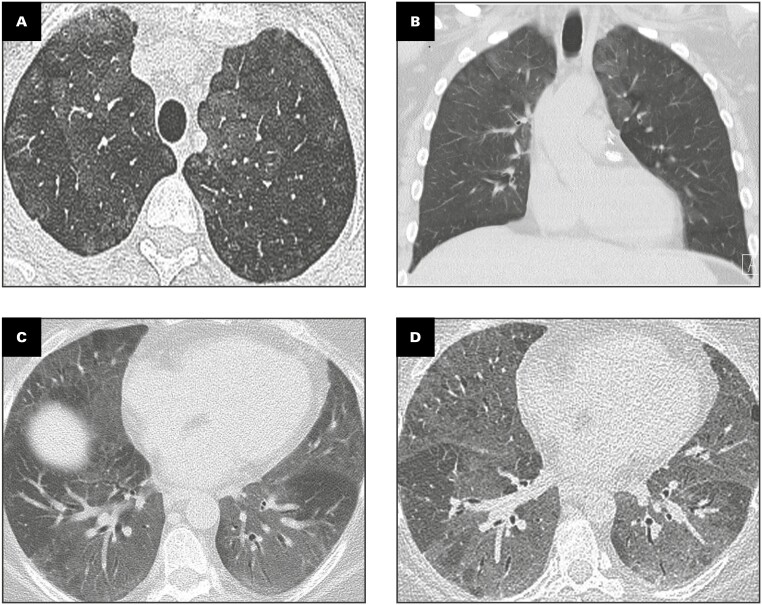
Radiologic findings in smoking-related interstitial fibrosis. A, B, Case 2 (44-year-old woman, 15 pack-year current smoker). High-resolution axial computed tomography (CT) (A) and nonenhanced coronal CT (B) demonstrate bilateral multifocal patchy ground-glass opacities in the upper lobes with bronchial wall thickening. C, D, Case 3 (48-year-old woman, 32 pack-year current smoker with forced vital capacity 63% predicted, diffusing capacity for carbon monoxide 33% predicted). Nonenhanced axial CT at diagnosis (C) and 89 months later (D) demonstrates diffuse ground-glass opacities in both lungs with bronchial wall thickening. Note stability of imaging abnormalities over more than 7 years.
